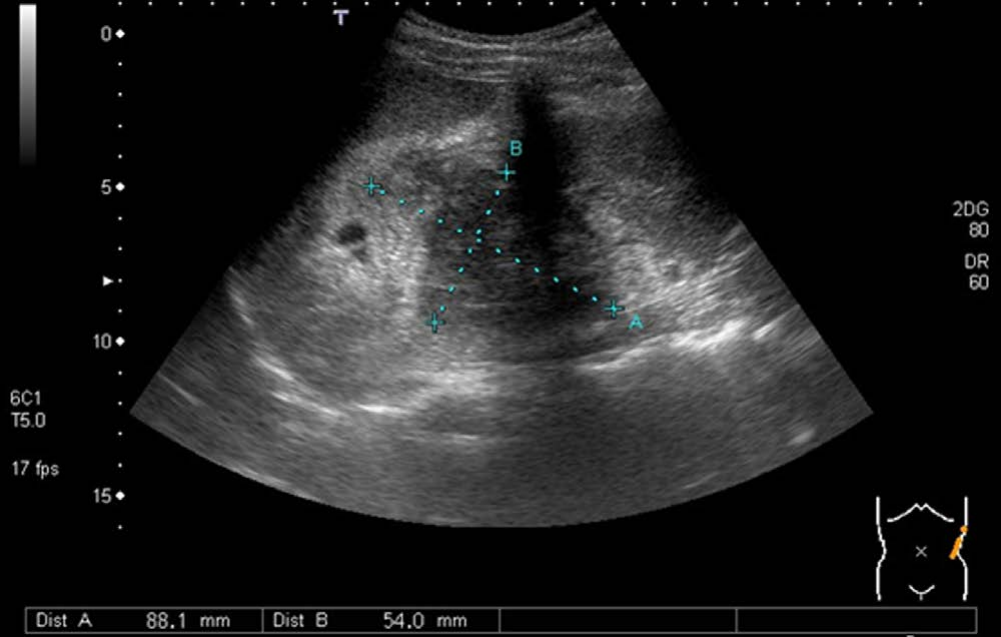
Abdominal ultrasonography. A heterogeneous mass (8.8 × 5.4 cm) was located between the spleen and left kidney with compression to the adjacent organs.
